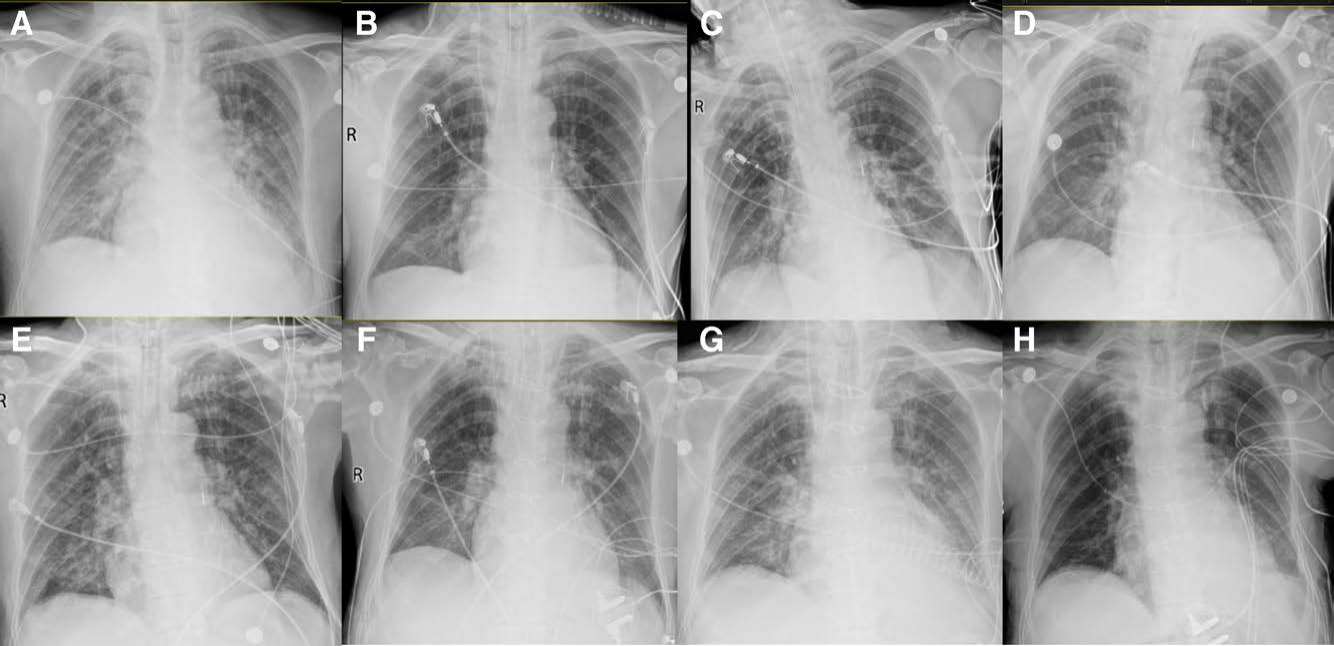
Chest x-ray images on (A) day 0: preintubation period following ICU admission; (B) day 6; (C) day 10; (D) day 16; (E) day 19: 24 hours preoperatively; (F) day 20: within 24 hours postoperatively; (G) day 22: the day following IABP discontinuation; and (H) day 28. IABP = intra-aortic balloon pump, ICU = intensive care unit.
